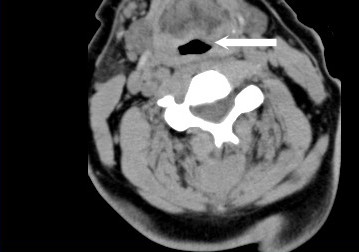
Axial reconstruction from a CT scan upon patient arrival show mild prominence of the prevertebral soft tissues, without clinically significant hematoma or encroachment on the airway (large white arrow).
